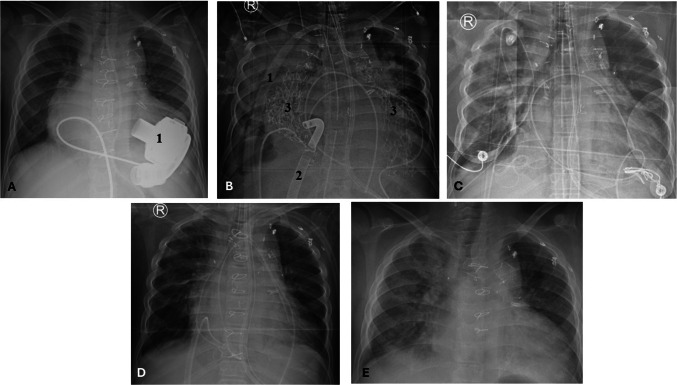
A Chest X-ray (CXR) showing HeartMate 3 ventricular assist device (1). B CXR showing Veno-arterial cannula (1—arterial, 2—venous) for extracorporeal membrane oxygenation (ECMO), QuickClot Gauze (3). C CXR post-ECMO decannulation and temporarily chest closure. D CXR post-definitive chest closure. E CXR 2 years post-heart transplant
